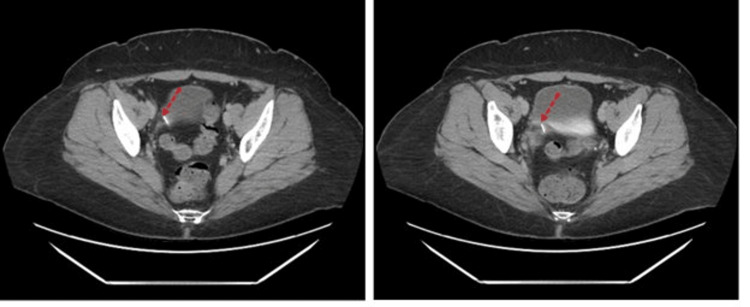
Computed tomography with contrast, axial plane. Catheter (red dotted arrows) of the gastric banding device coursing between the vagina and bladder at different levels.
